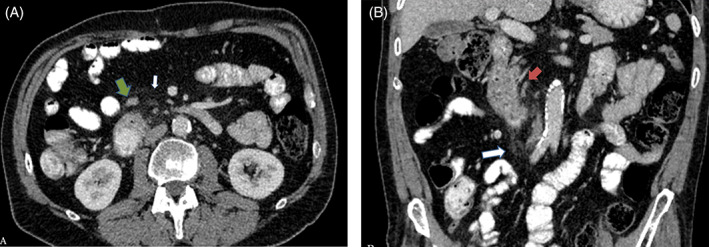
(A) Multidetector computed tomography (MDCT) with intravenous contrast in portal phase A. Axial. Periduodenal fat stranding (white arrow) and dilated local lymph nodes (green arrow). and (B) MDCT with intravenous contrast in portal phase B. Coronal. Periduodenal fat stranding (white arrow) and dilated local lymph nodes (green arrow), with the largest one (red arrow) measuring 7 mm
